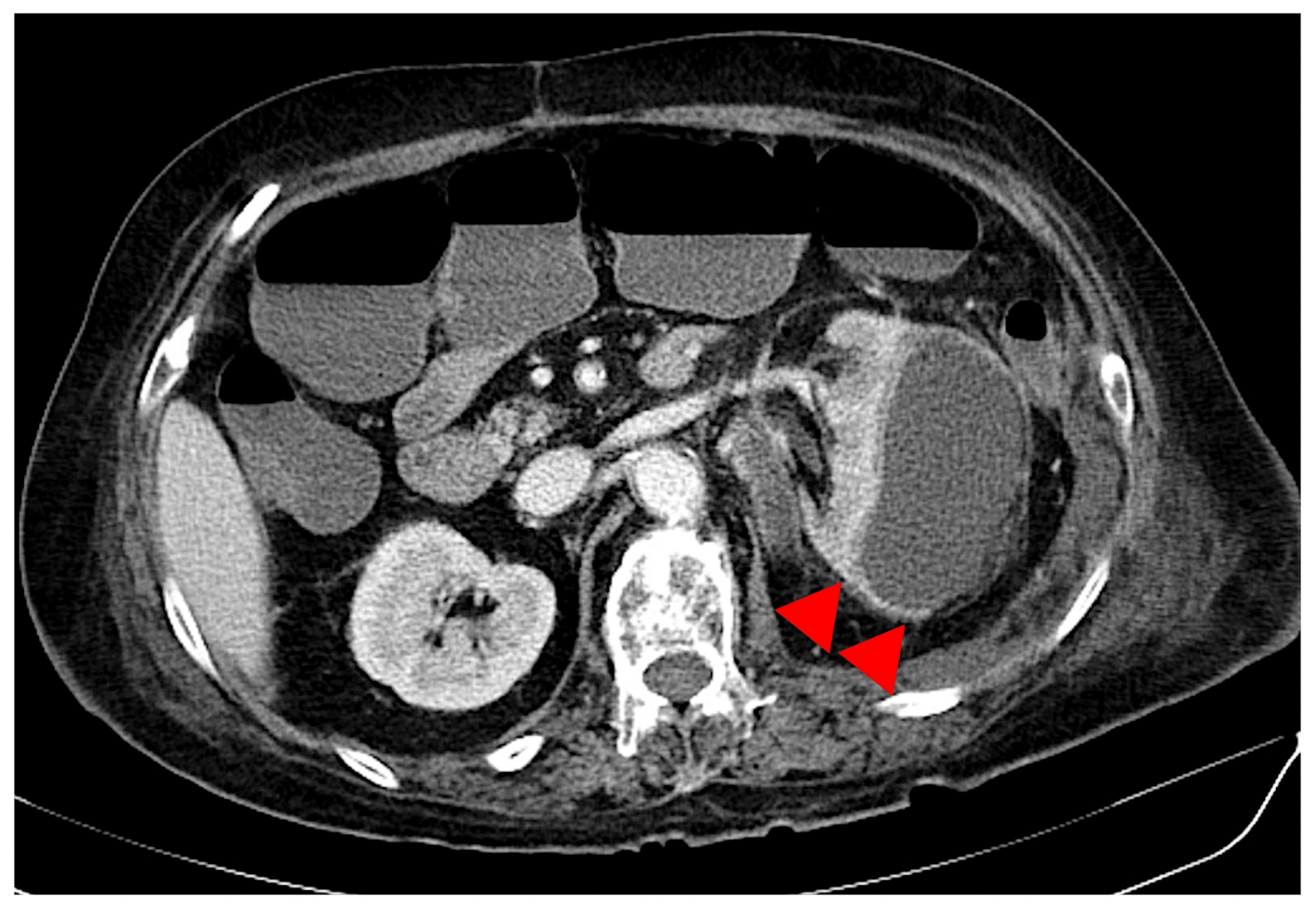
CT scan revealed a contrast effect suspicious for left subrenal capsular hematoma infection (red arrowheads).
